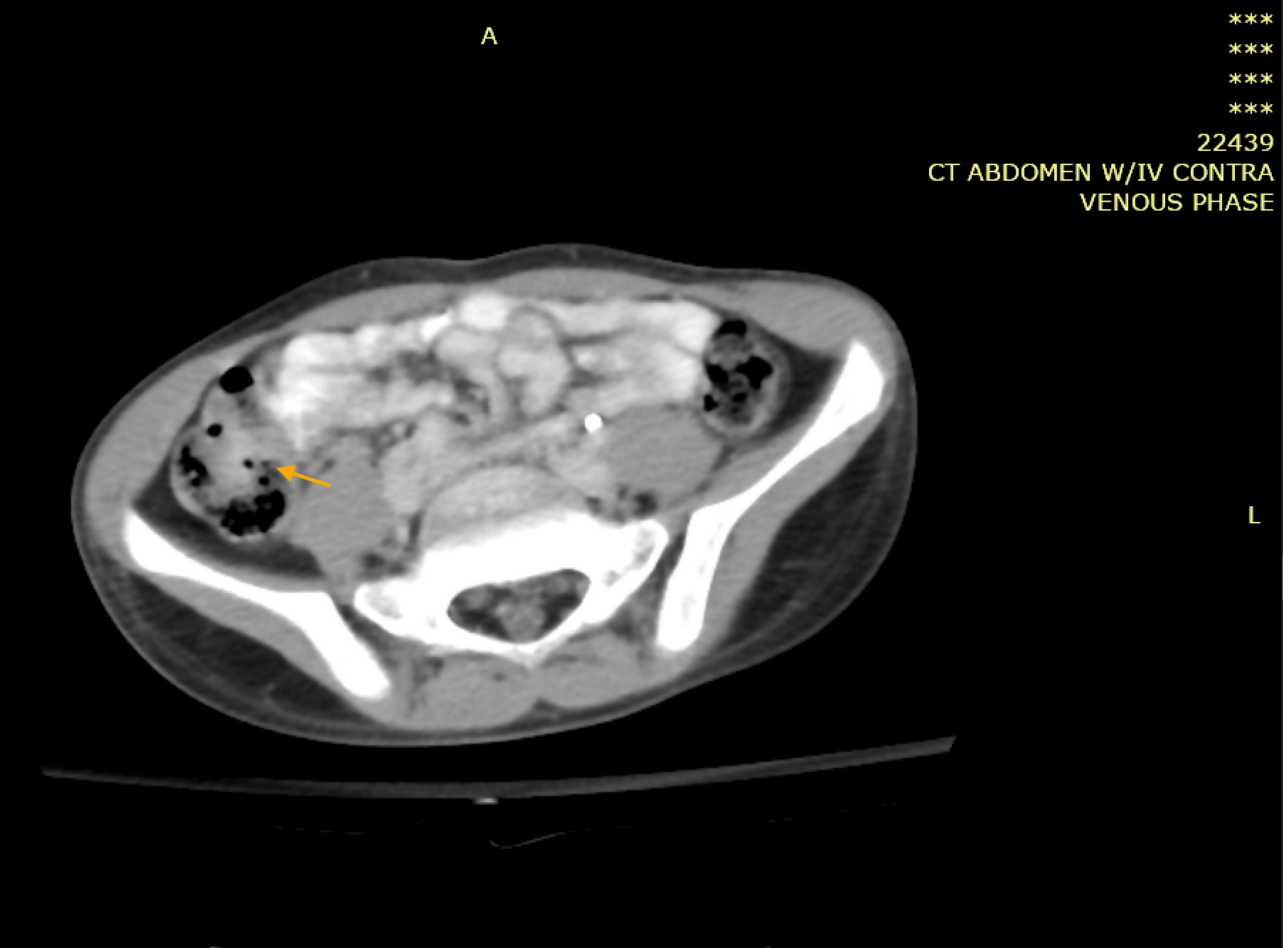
Computed tomography of the abdomen with IV contrast showing lymphadenopathy with almost complete resolution of the previously described wall thickening in the caecum and ascending colon region (orange arrow).
